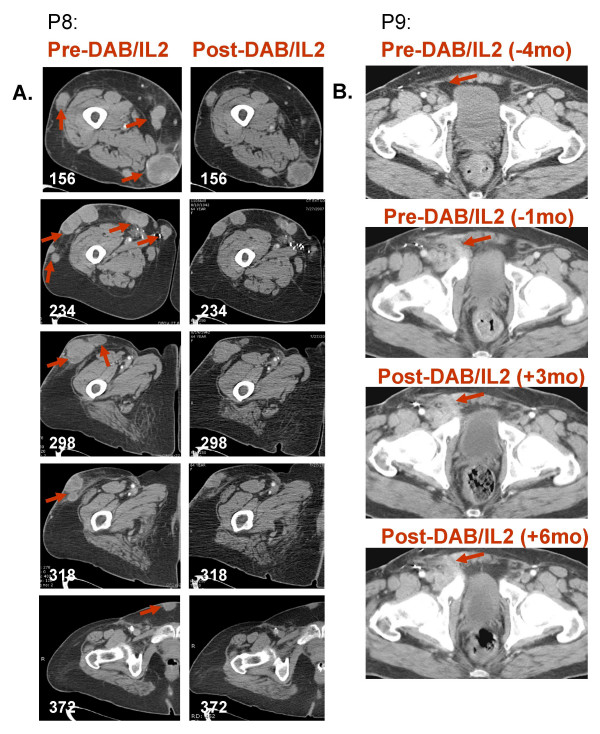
Regression of subcutaneous, intramuscular and lymphatic metastases after DAB/IL2 administration. A. The right lower extremity of patient P8 was scanned by CT imaging 3 weeks prior to DAB/IL2 administration (pre-DAB/IL2) and after completing four 3-week cycles of DAB/IL2 (post-DAB/IL2). The white numbers in the lower left corner of each image indicate the distance (mm) above the superior aspect of the patella in order to provide matched images for comparison. B. CT imaging of patient P9 revealed a rapidly growing right inguinal mass that decreased in size 3 months after DAB/IL2 administration. A follow-up scan, 6 months after DAB/IL2 administration, revealed no further growth.
